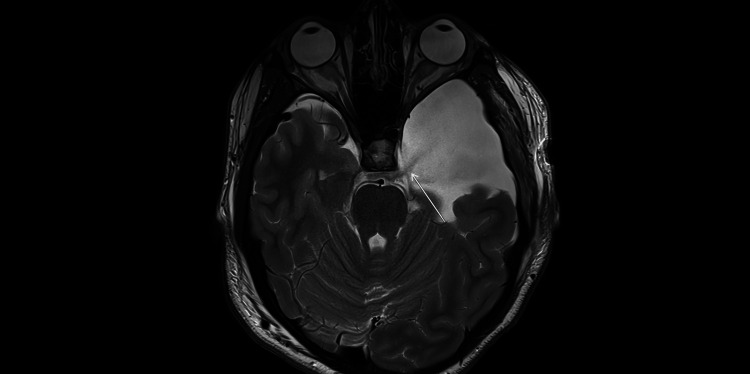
Early postoperative MRI (February 2025; axial T2-weighted; 4-mm slices; 0.5-mm gap). Left middle-fossa arachnoid cyst with a thin CSF-signal channel along its medial wall extending toward the basal cisterns (arrow), compatible with post-fenestration cyst-cistern communication.
